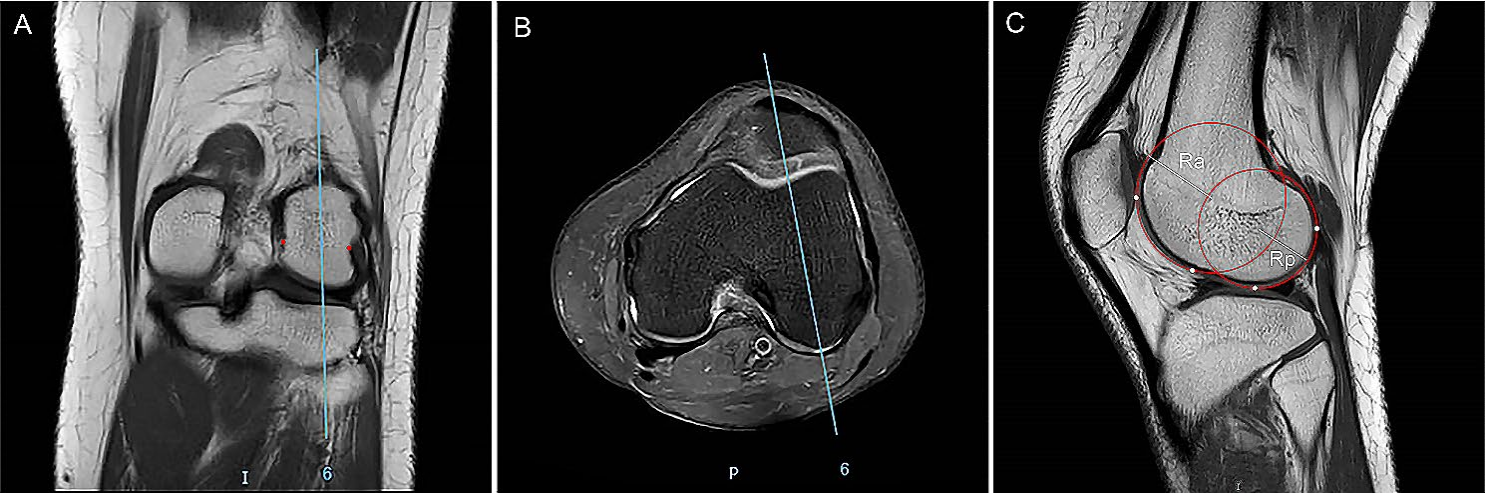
The measurement of lateral femoral condyle index (LFCI) by MRI. (A) MRI coronal plane with the most prominent popliteal groove is selected. (B) MRI axial plane showing the intact femoral trochlea and posterior femoral condyles is identified. (C) The relevant midsagittal plane is identified in combination with axial and coronal planes. The most inferior points of the anterior and posterior condylar cartilage, and the most anterior point and posterior point of the lateral condyle cartilage were marked (white dots). Two circles are drawn to the cartilaginous margin and pass through the marked points, respectively, to make each circle fit best with the spherical shape of the lateral femoral condyles. The LFCI is defined as the ratio of the radius of the posterior circle (Rp) and the radius of the anterior circle (Ra): LFCI = Rp/Ra
